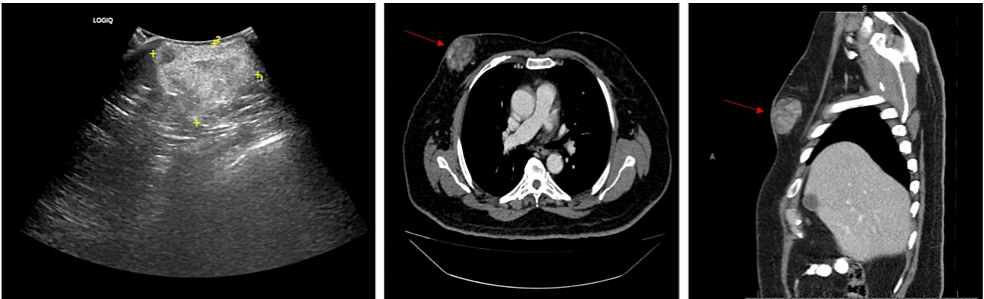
Diagnostic images (A) Ultrasound of right breast showing soft tissue lesion, (B) Axial computer tomography of chest showing soft tissue lesion (red arrow); (C) Coronal computer tomography of chest showing soft tissue lesion (red arrow).
